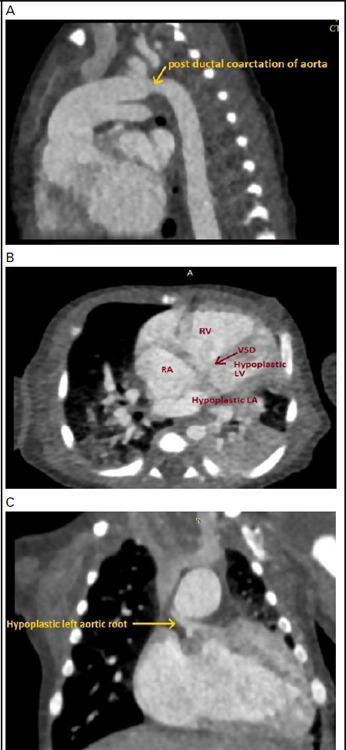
CT cardiac angio showing A) Post ductal coarctation of the aorta, B) hypoplastic left ventricle, dilated right ventricle and ventricular septal defect, C) hypoplastic left-sided ascending aortic root.
